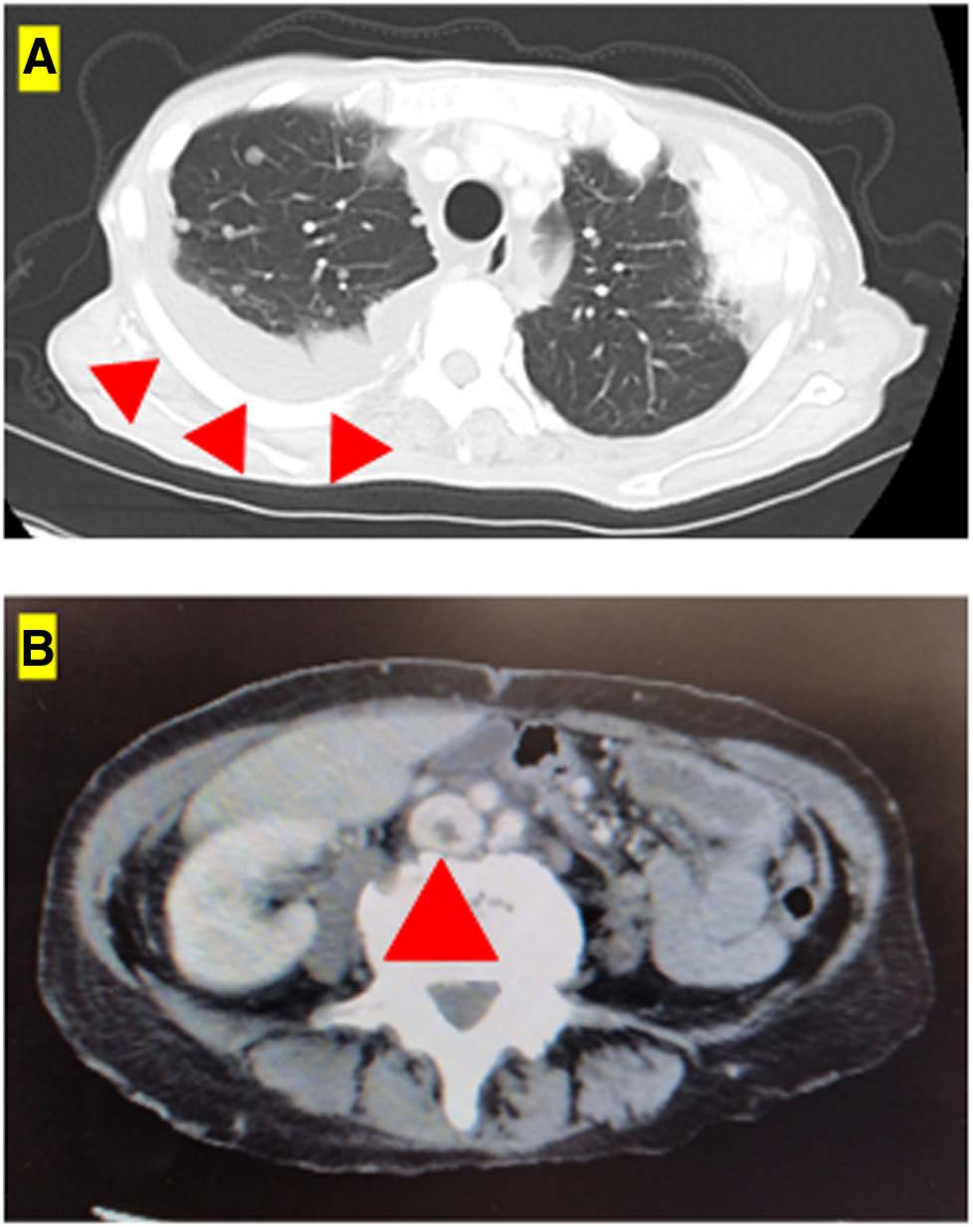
CT axial images show pleural effusion in the right lung field (A; red arrowheads) and thrombus in the inferior vena cava (B; red arrowhead).
